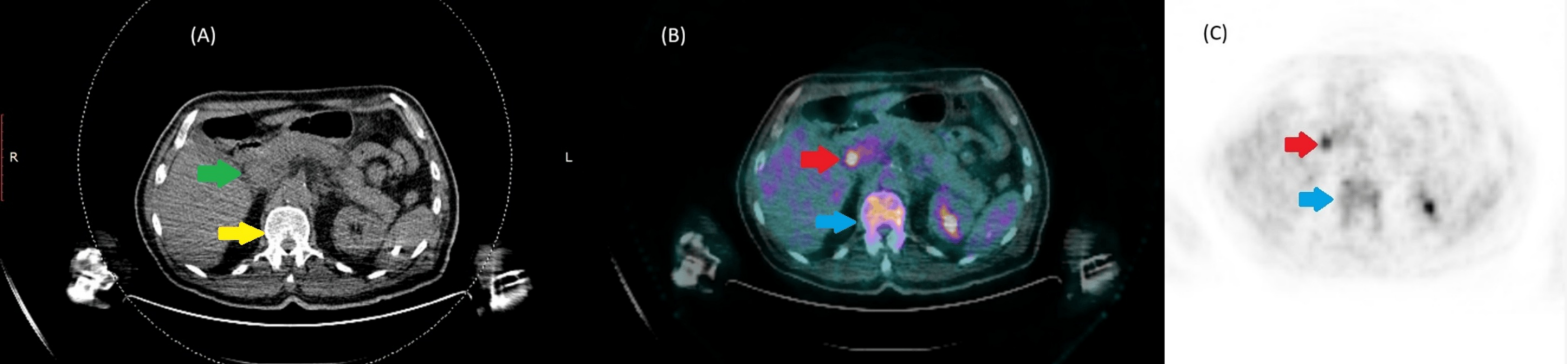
Axial PET-CT images of the abdomen showing hyper metabolism in the pancreas and bone marrow (A) Axial non-contrast CT image identifying the anatomical structures, including the head of the pancreas (green arrow) and axial bone marrow (yellow arrow), without focal mass or structural abnormality. (B) Fused PET/CT image demonstrating focus of increased FDG uptake at the level of the pancreatic head with SUVmax 8.3. (red arrow) and diffuse hypermetabolic activity within the bone marrow (blue arrow), suggestive of active inflammatory or hematopoietic involvement. (C) PET-only image highlighting the corresponding metabolic activity without anatomical detail: head of the pancreas (red arrow) and bone marrow (blue arrow) PET-CT: positron emission tomography-computed tomography; FDG: fluorodeoxyglucose; SUVmax: maximum standardized uptake value
